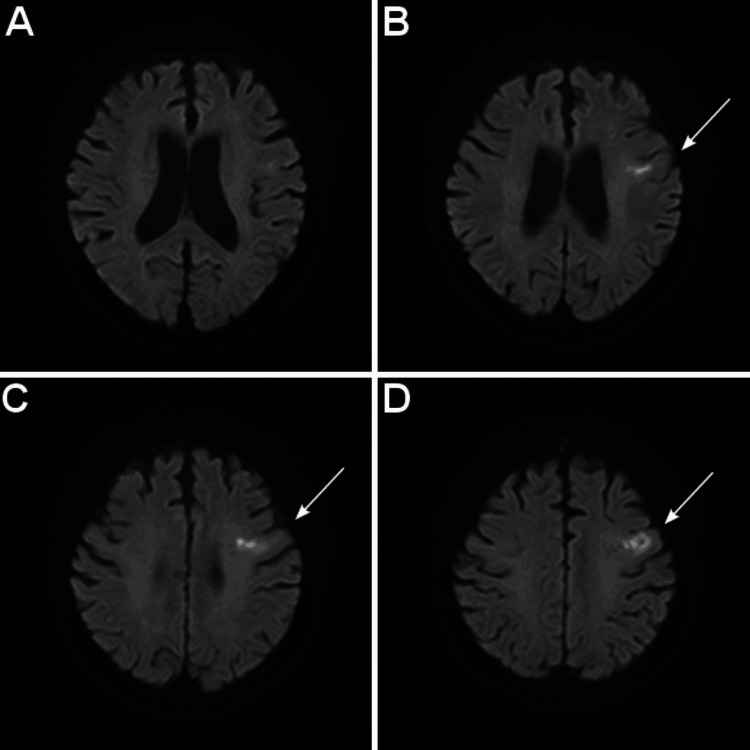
MRI a week after intravenous thrombolysis, revealing the peripheral middle cerebral artery territory infarction in the left precentral gyrus. Axial DWI (A–D). The arrow indicates an infarction. Note the sparing cortex of the left precentral gyrus.
